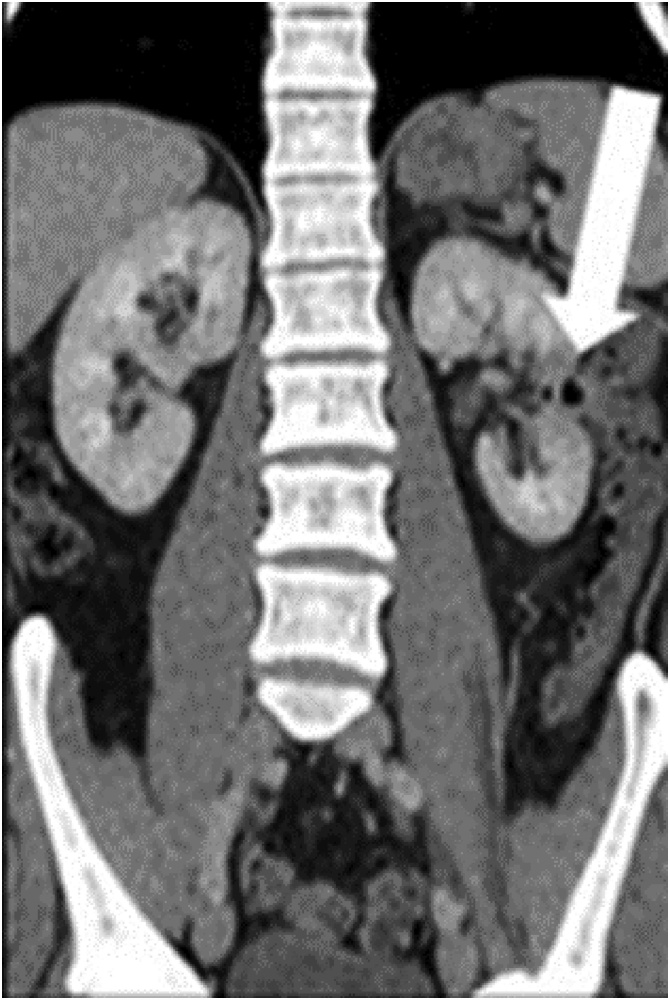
Abdominal CT with intravenous contrast. Left colorenal fistula after renal tumour cryotherapy.
